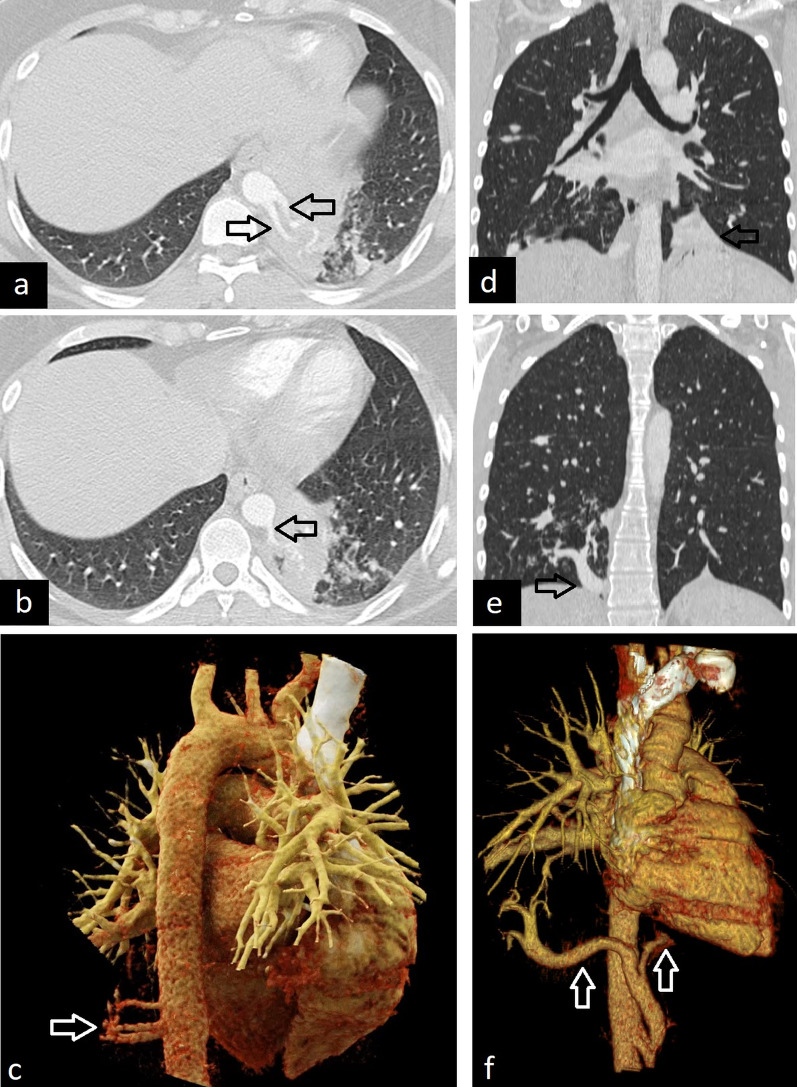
Different examples of pulmonary sequestrations. Axial CT images (a, b) and 3D cinematic volume-rendered reconstruction image (c) show three aberrant arteries which arise from the descending thoracic aorta and feed a consolidated area in the left lower lobe in a 25-year-old female with sequestration who presented with dyspnea (arrows). Coronal CT images (d, e) and 3D volume-rendered reconstruction (f) image demonstrate bilateral sequestrations with aberrant arteries arising from the abdominal aorta in a 22-year-old asymptomatic woman (arrows)
